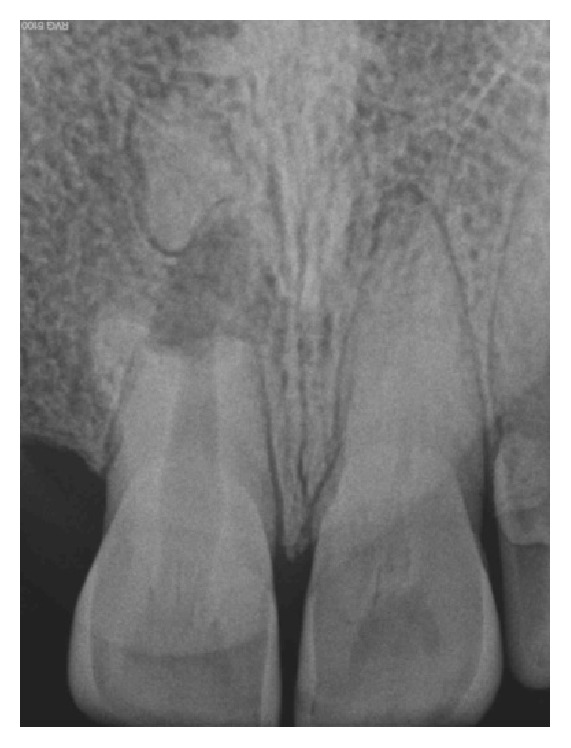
Preoperative intraoral periapical (IOPA) radiograph showing open apex in relation to 11.
